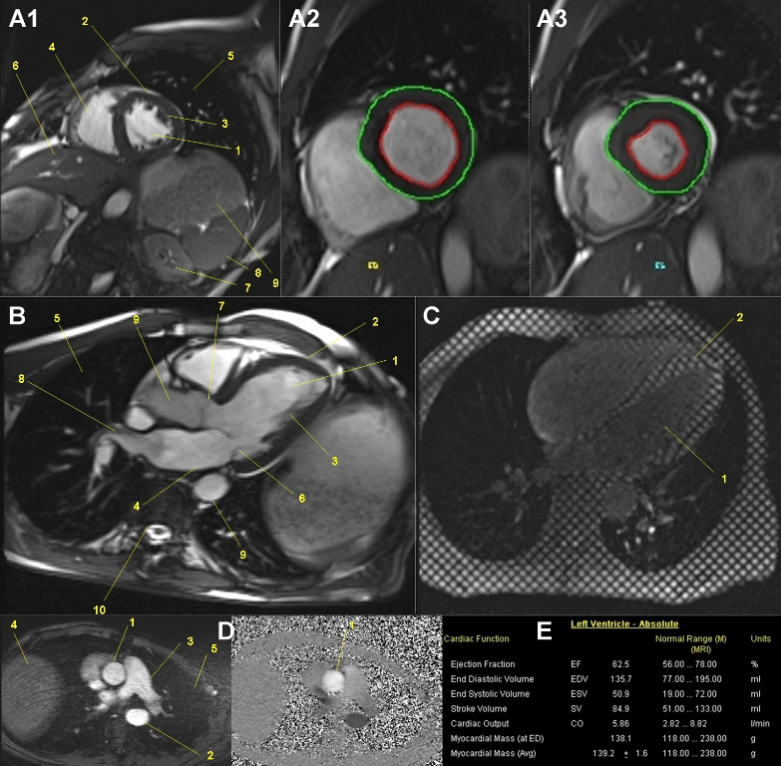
Mobile cardiac cine-MRI. A: male, 52-years-old, stage 23, 1,569 km (cine SSFP GRE, 2-chamber view): Focus is the left ventricle (1), myocardium (2). papillary muscles (3), right ventricle (4), lung (5), liver (6), left kidney (7), spleen (8), stomach (9). A2, A3: Specific post-processing makes functional volumetry of left ventricle and myocardium possible (green line: epicardium, red line: endocardium). B: male, 52-years-old, stage 25, 1,706 km (cine SSFP GRE, 3-chamber view): left ventricle (1), myocardium (2), papillary muscles (3), left atrium (4), lung (5), mitral valve (6), aortic valve (7), pulmonary vein (8), aorta (9), thoracic spine (10). C: male, 49-years-old, stage 26, 1,770 km (cine tagging SSFP GRE, four-chamber view): MR tagging of the left ventricle (1) makes quantification of the myocardial (2) motion with its spatial orientation possible. D: female, 45-years-old, stage 38, 2,601 km (phase contrast transversal): Ascending aortic (1) flow is measured by specific velocity-encoded (venc) MR imaging. Descending aorta (2), pulmonary artery (3), liver (4), lung (5). E: Selection of possible cardiac parameters measurable by cardiac cine-MRI.
